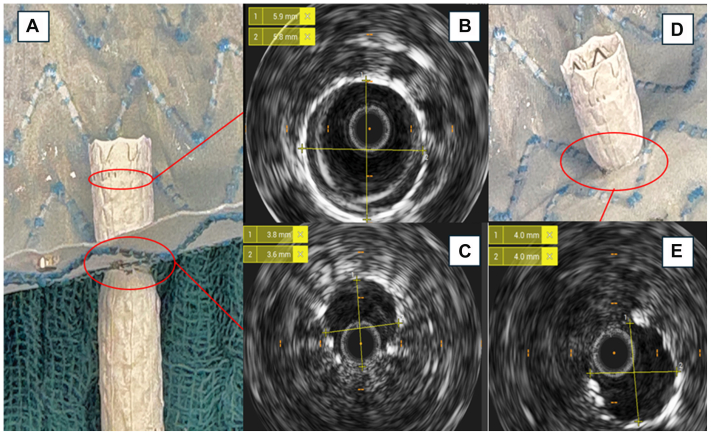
Bridging stent (BS) graft expansion measure by mean of intravascular ultrasound in the Endurant IIs graft after plain ballooning only. Implanted BS graft before flaring (A) and relative diameter measurements outside the fenestration (B) and at the fenestration level (C). Stent after flaring (D) and diameter measurement at fenestration level (E).
